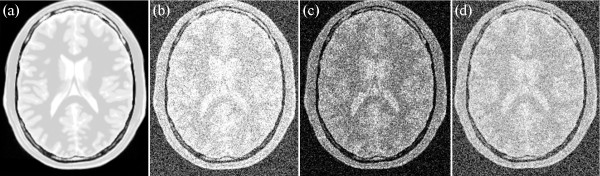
An example of PD image: (a) original PD weighted MRI, (b) 17% noisy PD weighted MRI, (c) squared magnitude of the noisy PD weighted MRI, (d) the forward variance-stabilization transformation of the noisy PD weighted MRI.
